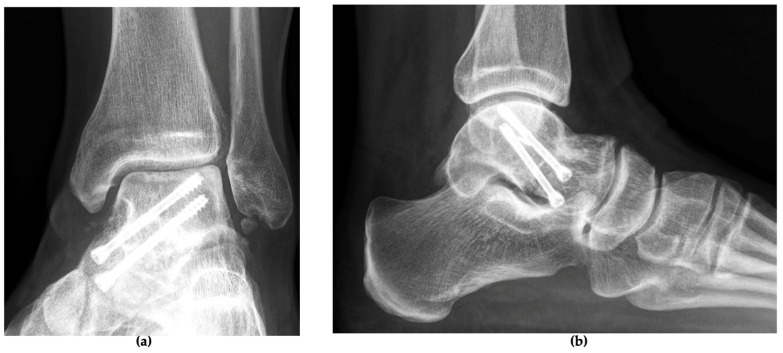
Radiographic imaging at the two-year follow-up in (a) AP view and (b) lateral view, showing a healed left ankle with restored anatomy.
